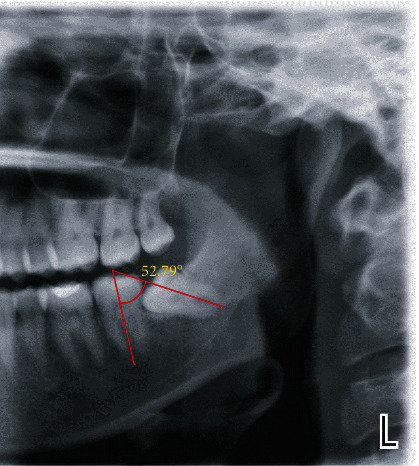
Measurement of angulation of third molar on panoramic radiograph.
